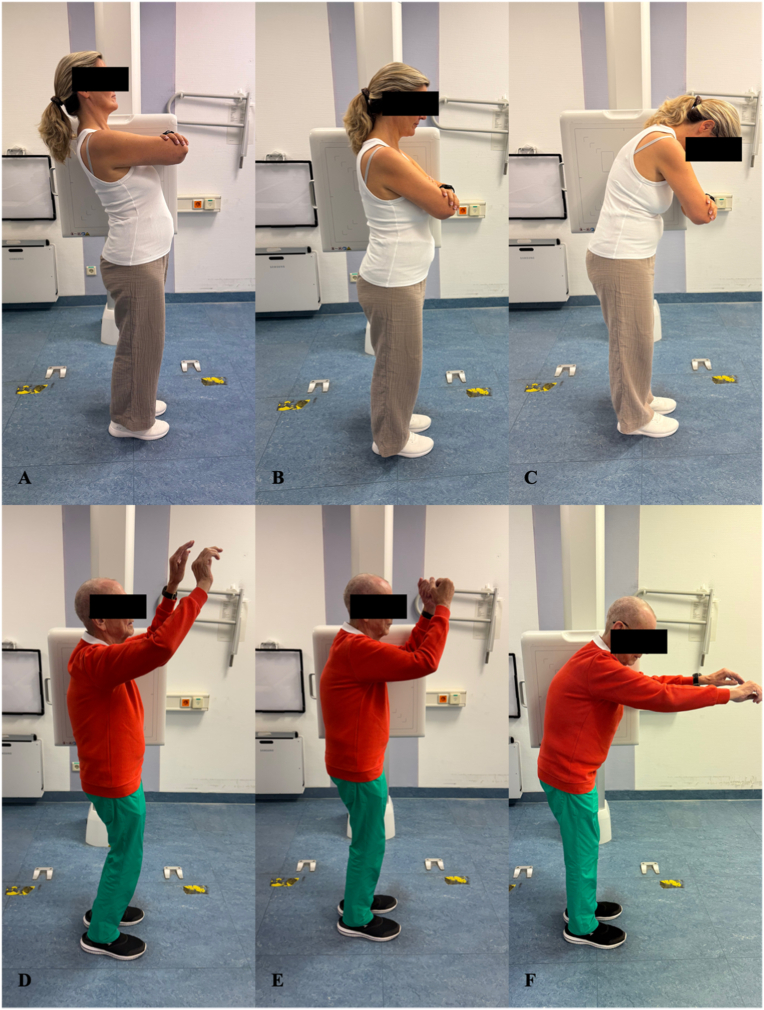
Impact of lumbar mobility on flexion-extension radiographs. Neutral (B, E), flexion (C, F), and extension (A, D) lateral positioning during flexion-extension radiographs in two patients with preserved (A-C) versus impaired (D-F) lumbar mobility. Limited lumbar motion in the second patient highlights the difficulty of performing dynamic flexion-extension imaging in cases of restricted lumbar flexibility.
